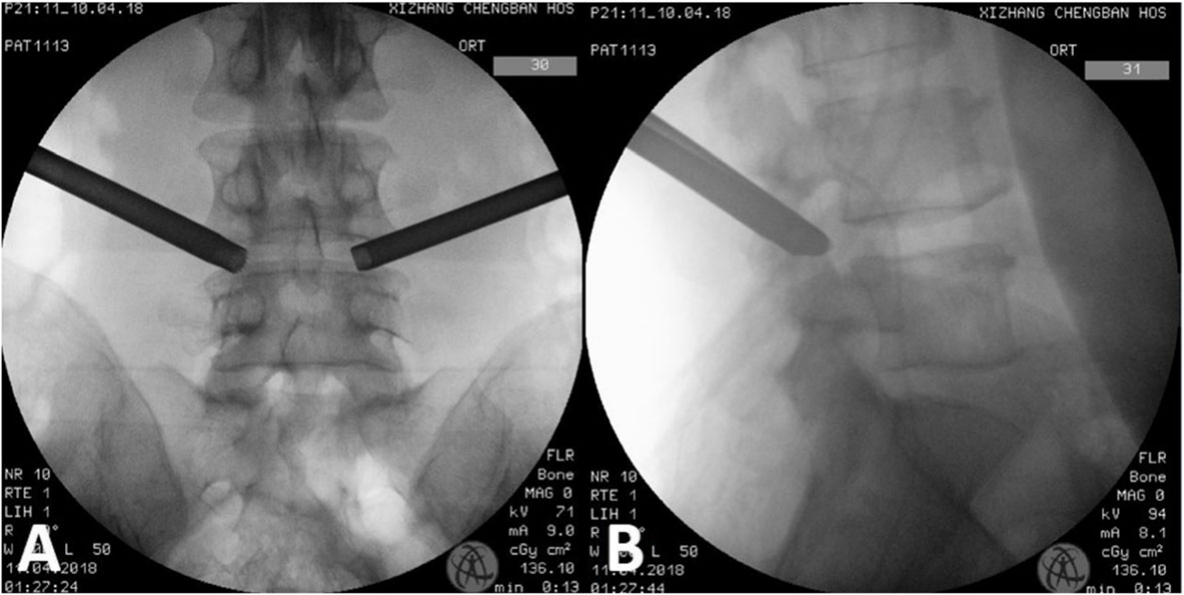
The working channel position of bilateral foraminoplasty. a X-ray anteroposterior view, b X-ray lateral view
